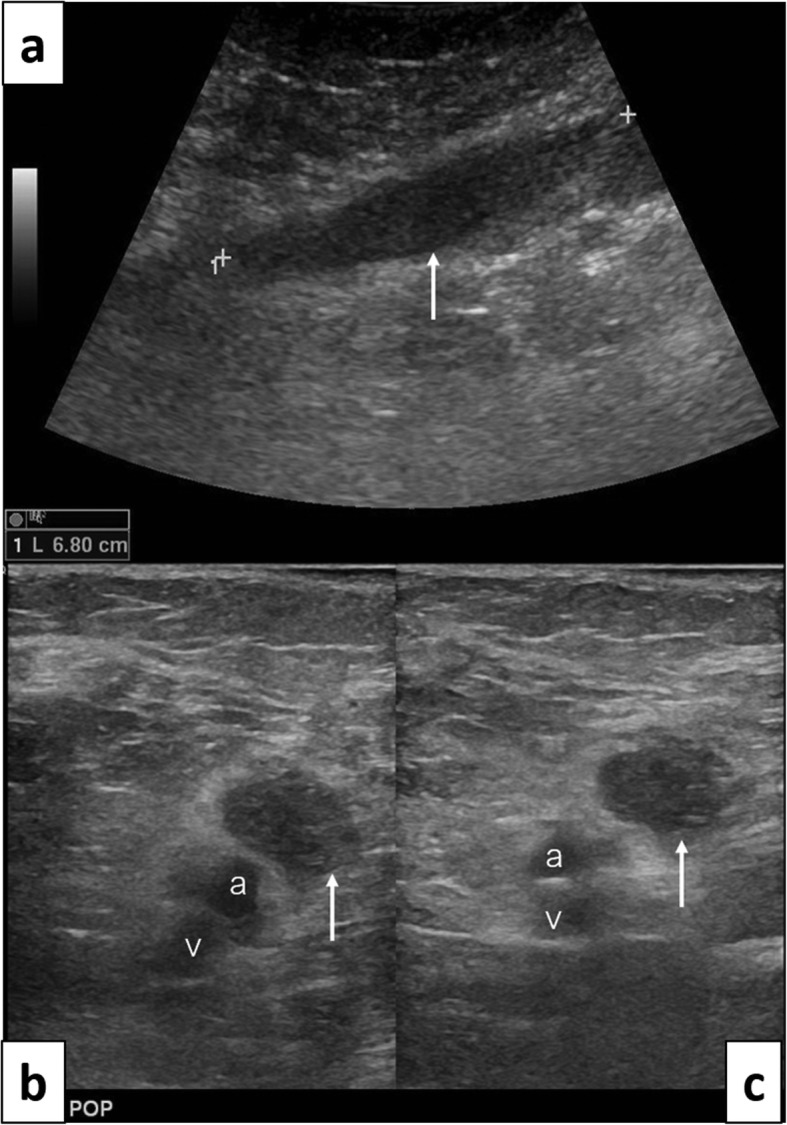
Case 3. 67 years old woman. Ultrasound of the distal thigh/popliteal fossa. a Long axis image with convex probe demonstrating a hypoechoic elongated oval-shaped longitudinally oriented mass (arrow). This corresponded to the course of the sciatic nerve. b and c Short axis view of the same lesion with high resolution 12 MHz linear probe without and with probe compression, confirming the presence of a non-compressible hypoechoic lesion (arrows), adjacent to the popliteal artery (a) and vein (v). Note the partial collapse of the popliteal vein (v) in (c)
